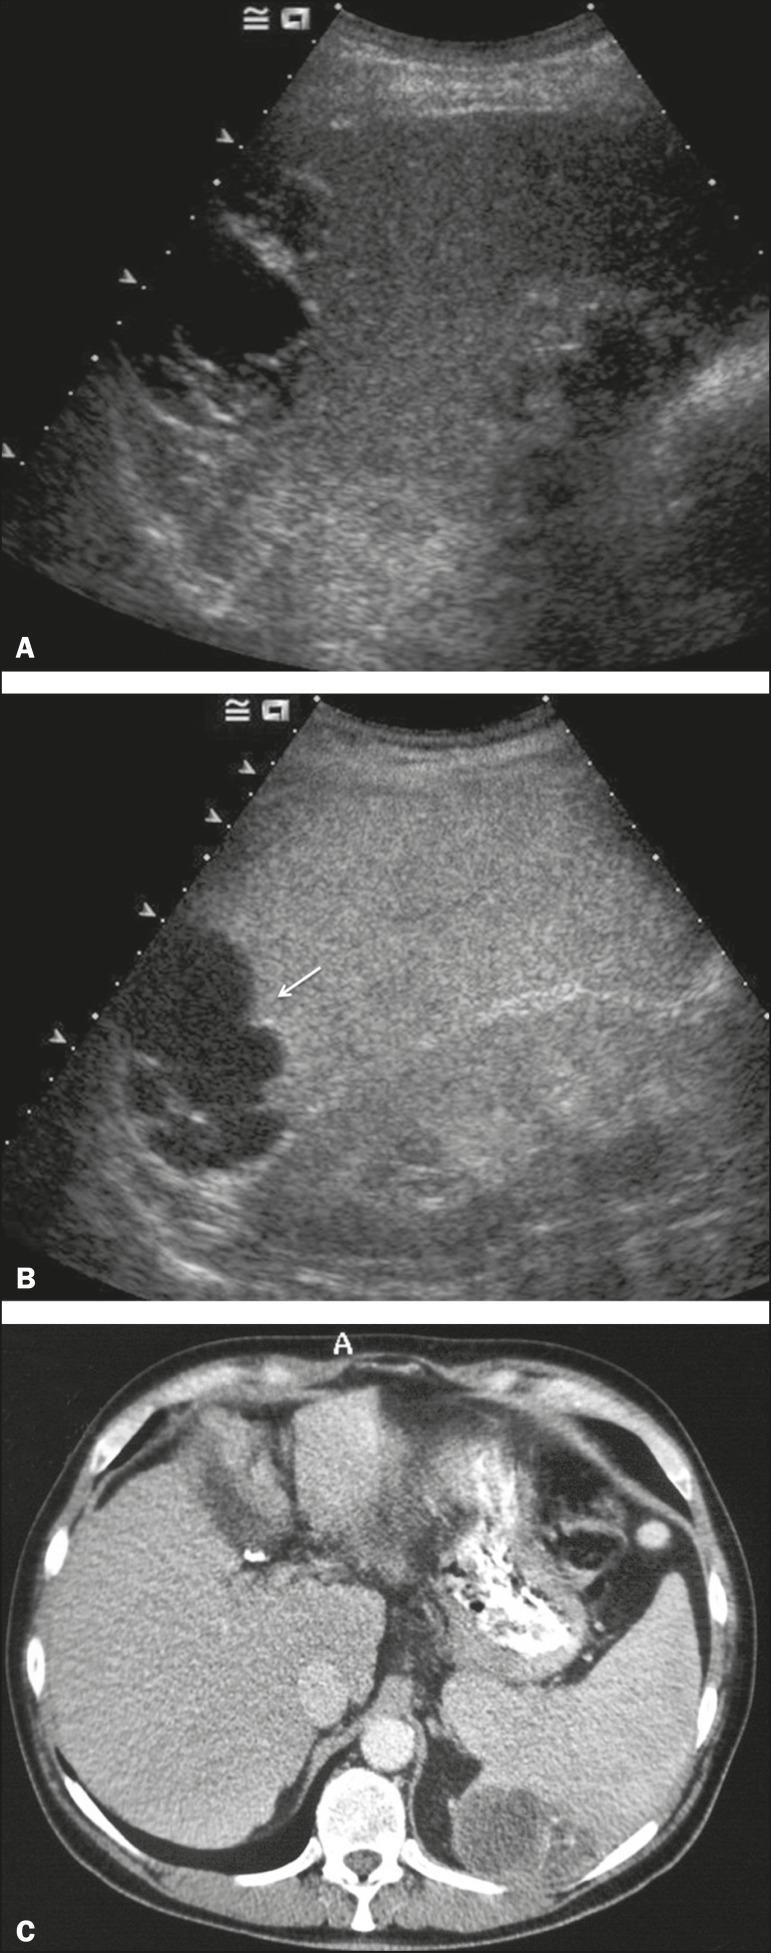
Splenic lymphangioma. A: B-mode ultrasound showing a
